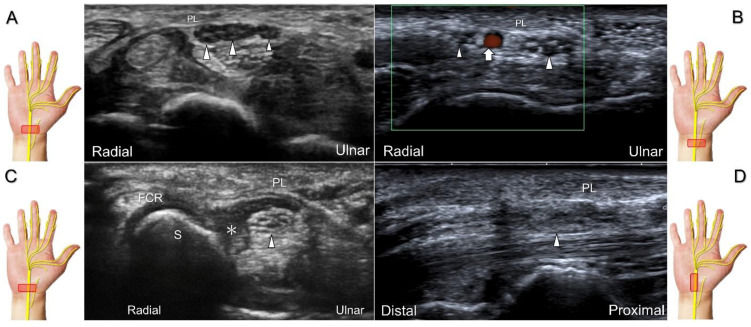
Sonographic images show a bifid median nerve (A), a persistent median artery with a bifid median nerve (B), accessory flexor digitorum superficialis muscle (C), and laceration of the palmaris longus (PL) tendon with the compression of the median nerve (D). White arrowheads: median nerve; white arrow: persistent median artery; asterisk: accessory flexor digitorum superficialis muscle. FCR: flexor carpi radialis tendon; S: scaphoid.
